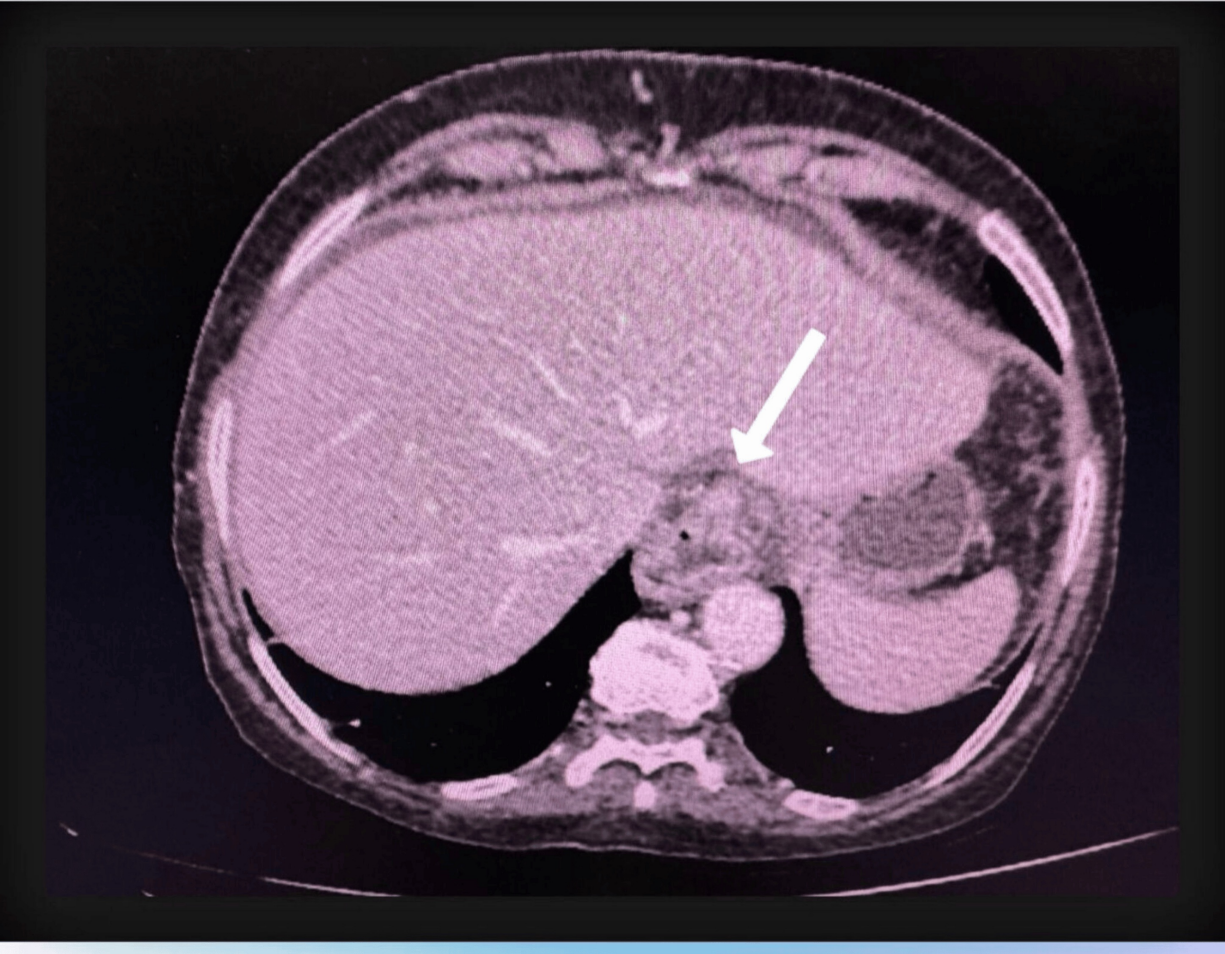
CECT showing irregular thickening of distal esophagus (arrow) CECT: contrast-enhanced computed tomography
